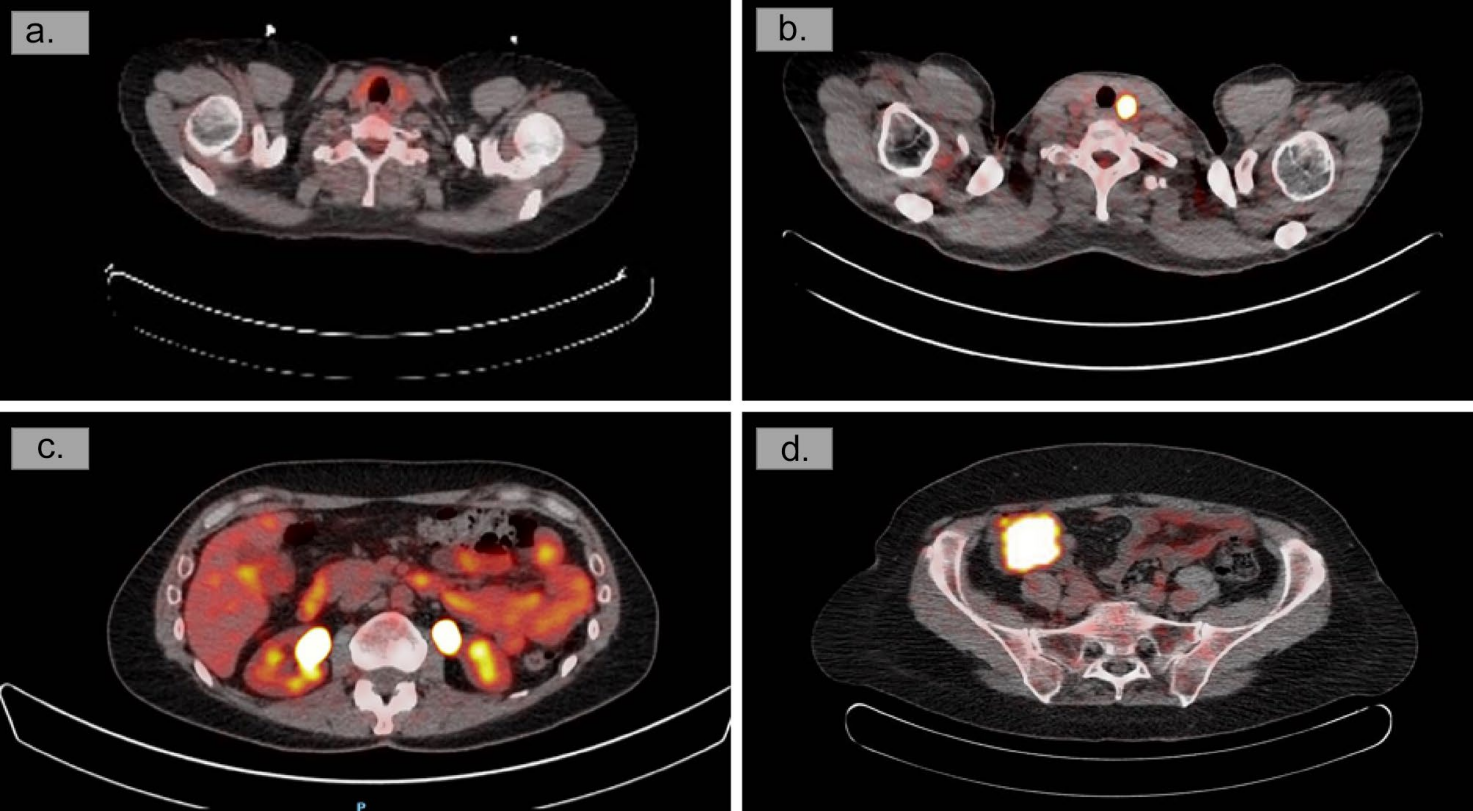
Malignant and benign additional non-BC-related findings. A benign multinodular thyroid lesion (a) compared to a papillary thyroid carcinoma with focal uptake (b) and a gastro-intestinal lesion with diffuse FDG uptake without any abnormality on colonoscopy (c) compared to a colon carcinoma (d)
